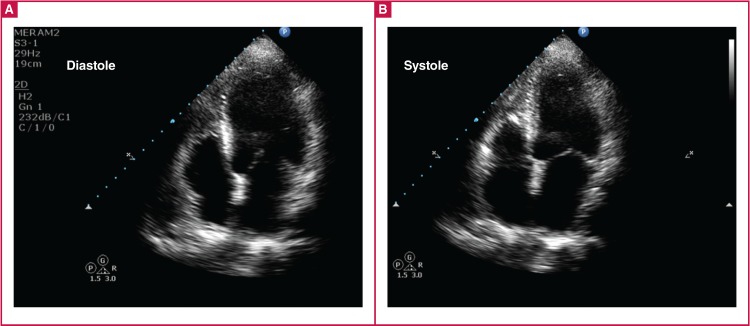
After warfarin treatment, echocardiography showing improved left ventricle and resolved thrombus in the diastolic and systolic phase.
